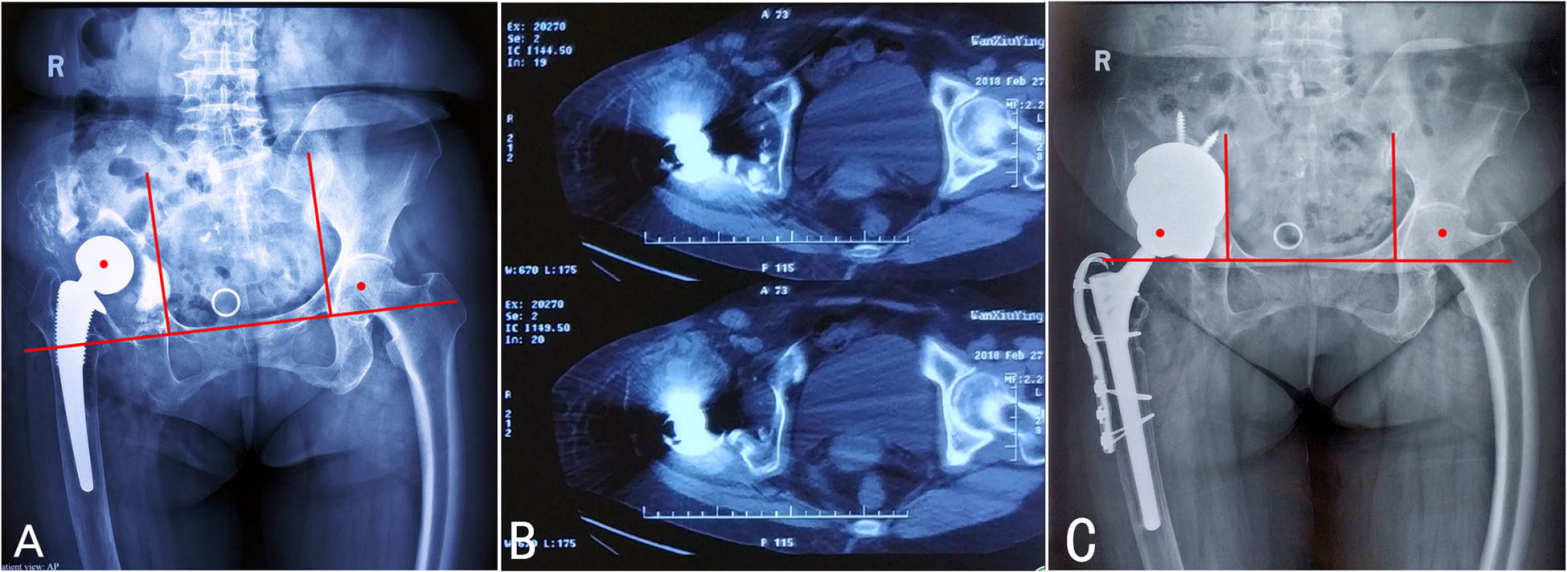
A 66-year-old female patient who had an infected total hip arthroplasty. A The preoperative X-ray showing a Paprosky IIIB defect. B The pelvic continuity was confirmed on CT scans. C The “hybrid cup” reconstruction technique was used with 3 porous shells. The smaller middle cup was eccentrically cemented into the medial TM revision shell to fill the sideward defects. A larger porous cup was then impacted onto the “cup-in-cup” construct (out diameters of the shell from medial to lateral: 66 mm; 54 mm; and 62 mm)
